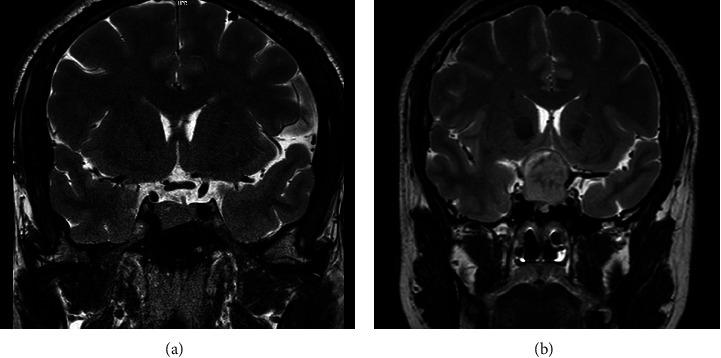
MRIs of GH-secreting PitNETs. (a) T2-weighted MRI of a patient with densely granulated somatotroph tumor. Tumor size 1.7 cm × 1.6 cm × 1.1 cm (arrow). (b) T2-weighted MRI of a patient with sparsely granulated somatotroph tumor. Tumor size 2.5 cm × 2.6 cm × 2.7 cm (arrow). MRI, magnetic resonance image; GH, growth hormone; PitNETs, pituitary neuroendocrine tumors.
